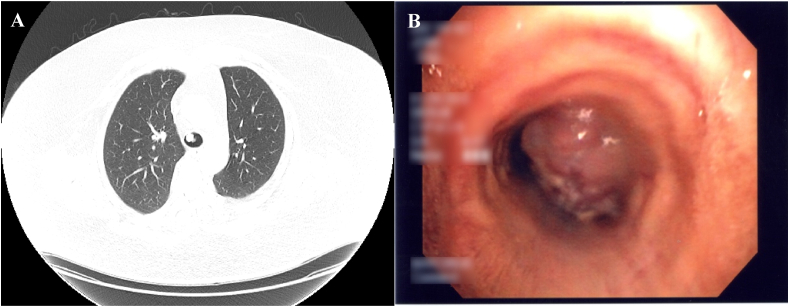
(A) Axial computed tomography of the thorax with lung windows demonstrates an intraluminal anterior tracheal mass. (B) Tracheal mass visualized on flexible bronchoscopy.
